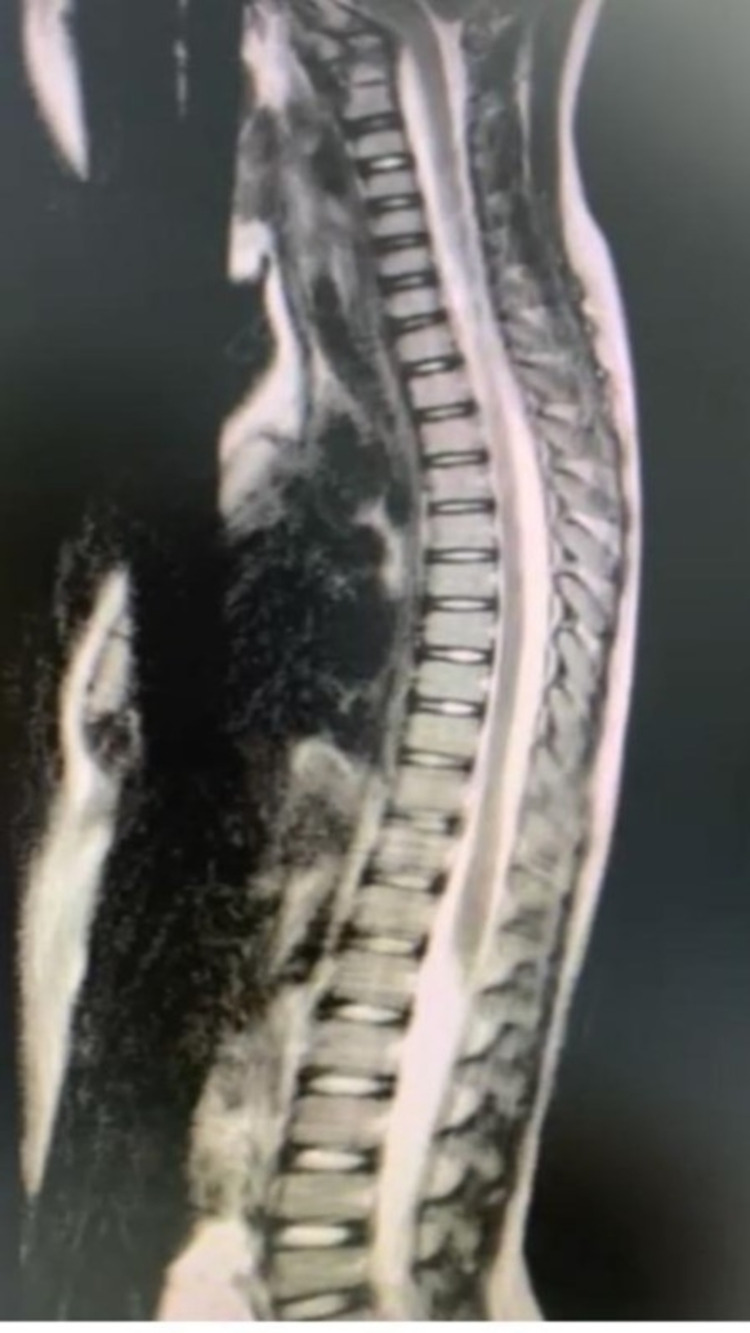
Postoperative image - MRI whole spine
